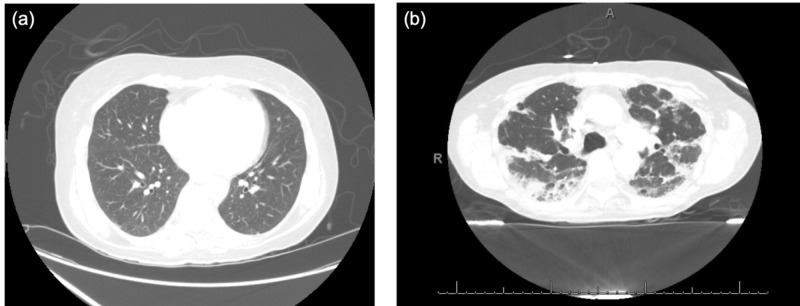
High-resolution computed tomography of the chest for patient 1. (a) High-resolution computed tomography of the chest for patient 1 prior to the initiation of mFOLFOX-6, (b) High-resolution computed tomography of the chest for patient 1 after 15 cycles of mFOLFOX-6 demonstrates extensive mixed solid and ground-glass opacities with mild bronchiectasis.
